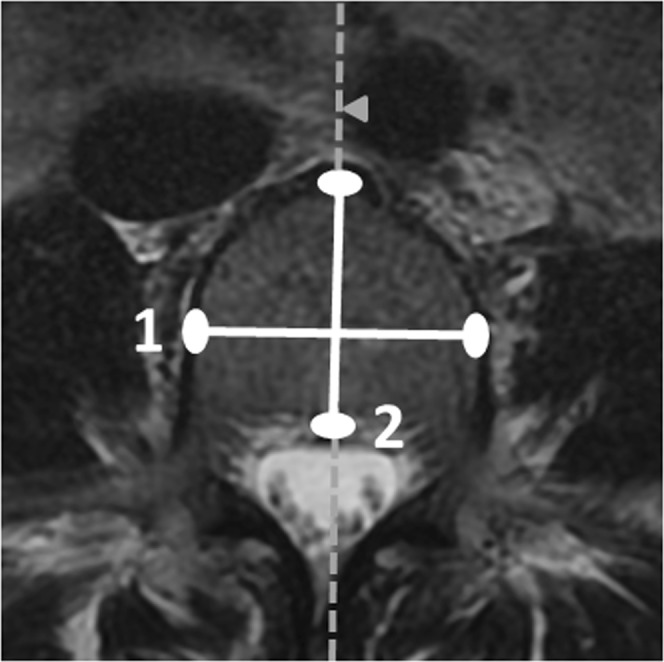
Annotated axial MRI scan of L4. Vertebral width = Measurement 1 and vertebral depth = Measurement 2. After all axial planes were examined, the maximum and minimum widths and the superior, inferior and midway depths were recorded.
